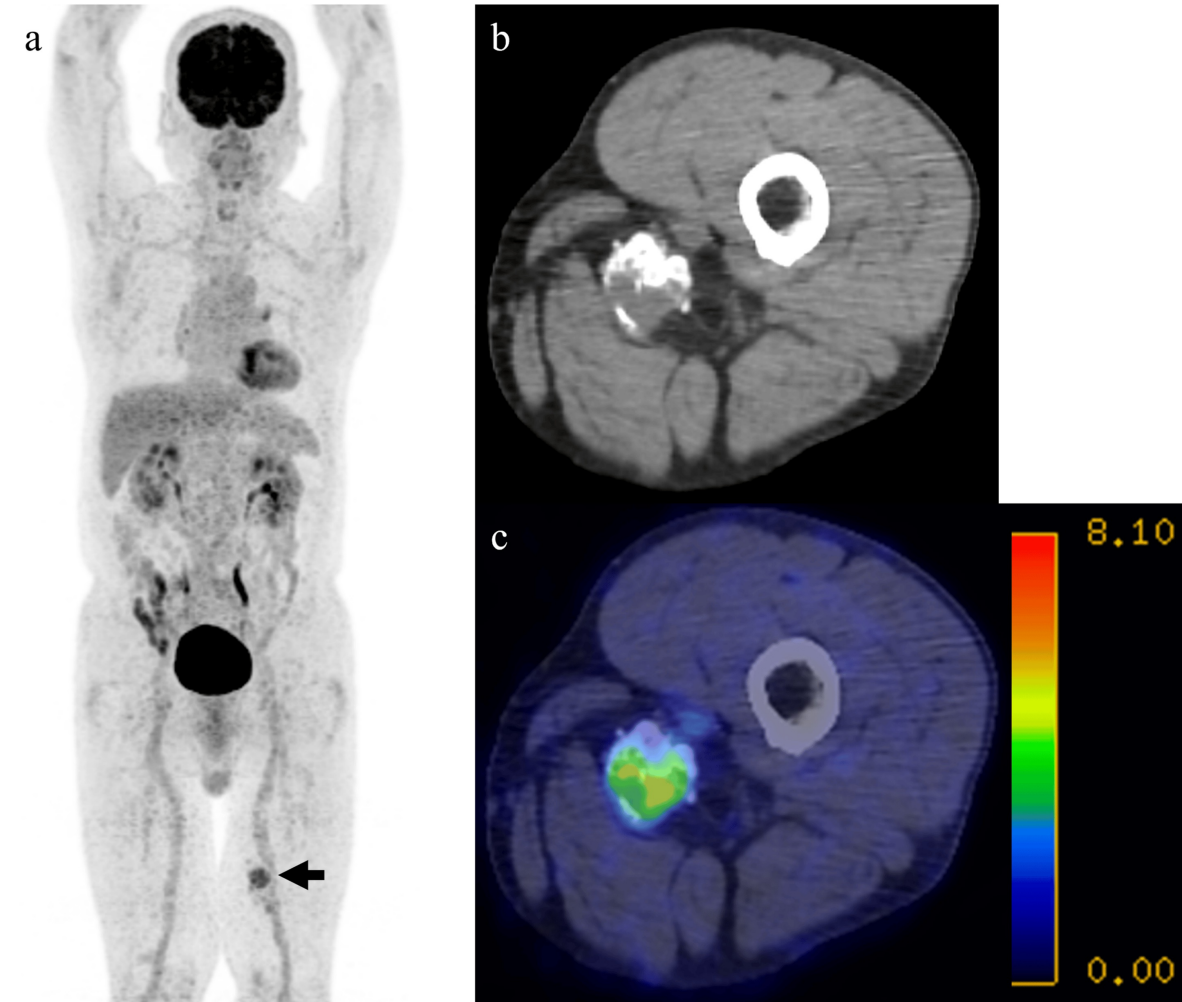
PET/CT images evaluated for suspected primary lung cancer in an asymptomatic 80-year-old male MIP image showing increased FDG uptake in the left thigh (a). Attenuation-correction CT (b) and fused PET/CT image (c), demonstrating a SUV max of 5.6. PET/CT: positron emission tomography/computed tomography, FDG: fluorodeoxyglucose, SUV max: maximum standardized uptake value, MIP: maximum intensity projection
